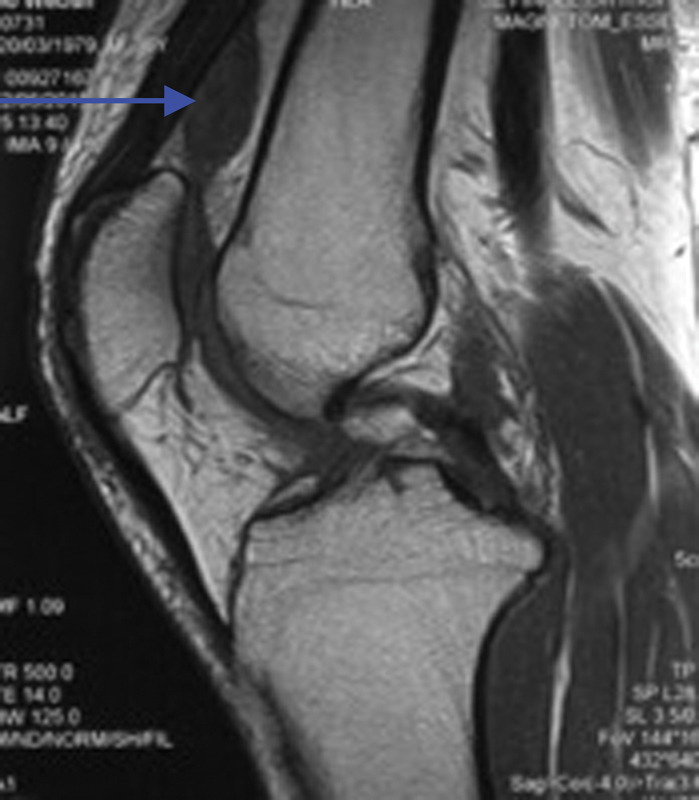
Suprapatellar mass which was hypointense in T1-weighted image (blue arrow).
